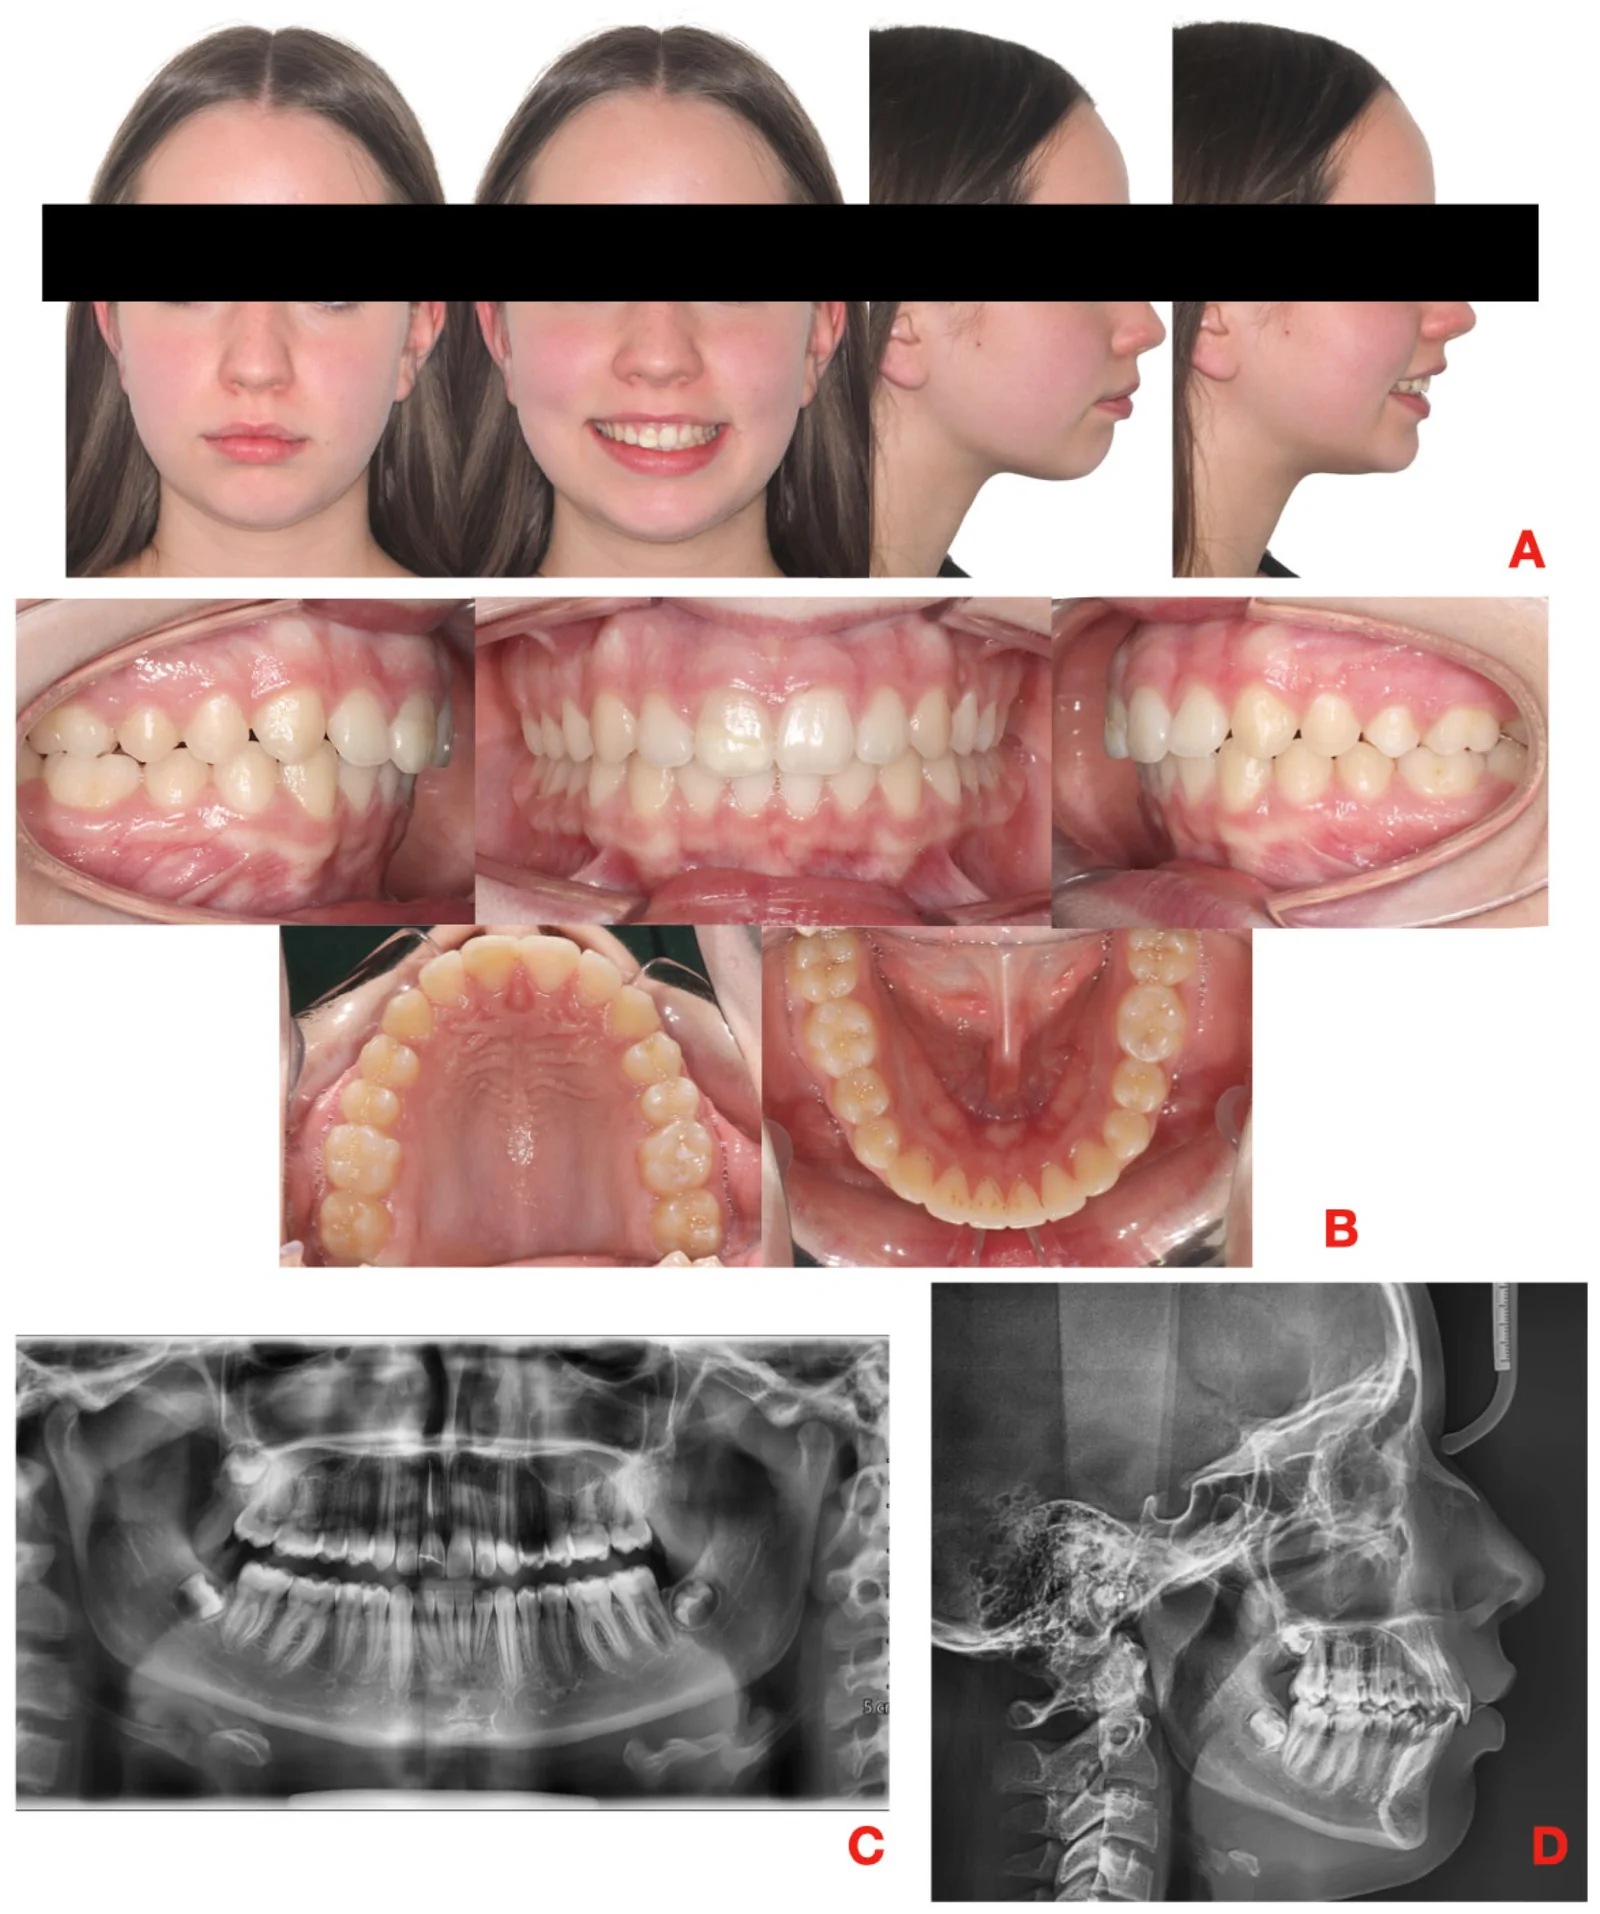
Final records of the 11-year-old female patient at the end of treatment (T1). (A). Extra-oral photographs in frontal and lateral views. (B). Intra-oral photographs in frontal, lateral, and occlusal views. (C). Orthopantomography. (D). Lateral cephalogram radiograph.
